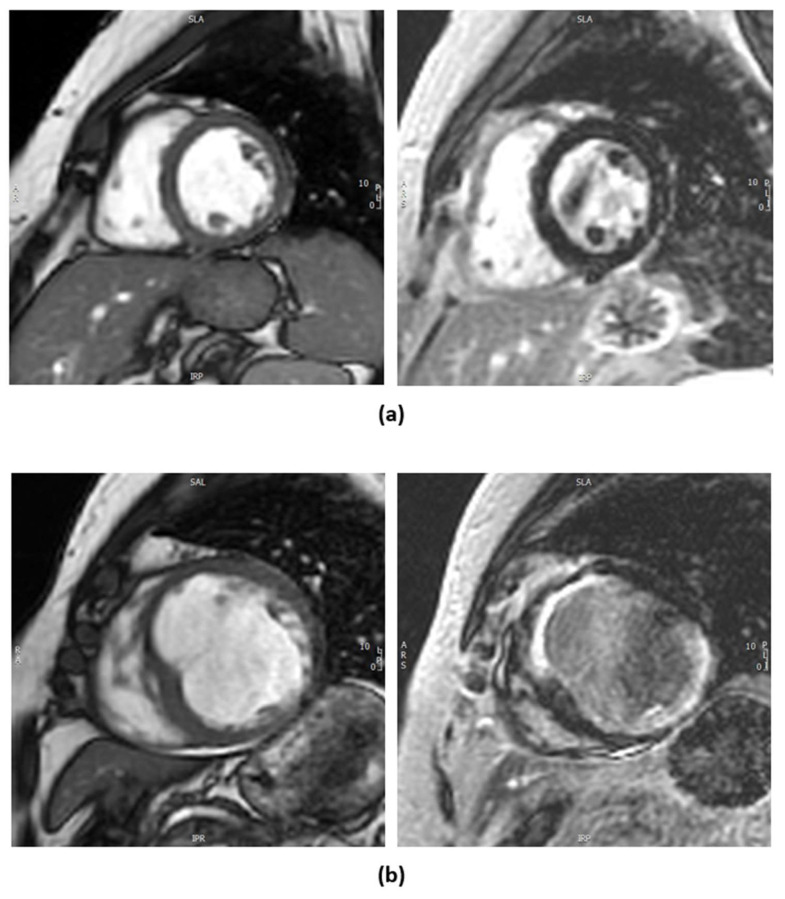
Contrast cardiac MRI with gadolinium contrast. (a) Left image shows a 2D cine image clip of mid-ventricle short axis of a patient with ischemic cardiomyopathy. The image to the right corresponds to post-gadolinium contrast imaging showing no late gadolinium enhancement (LGE) indicating viable myocardium. (b) Left image shows a 2D cine image clip of mid-ventricle short axis of another patient. There is thinning of the anteroseptal, anterior, and inferolateral segments. The right image corresponds to post-gadolinium imaging with full-thickness LGE in the anterior, anteroseptal, and inferolateral segments indicating non-viable myocardium in the left anterior descending artery (LAD) and left circumflex (LCx) territories.
